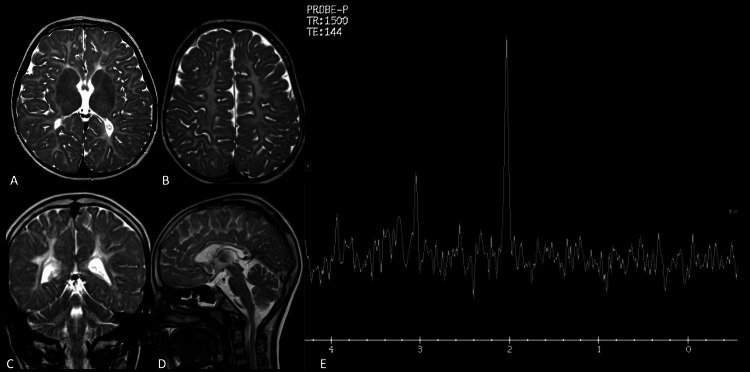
Salla's disease Confluent white matter T2 hyperintensity is visualized above (A) and at the level of the basal ganglia (B). The subcortical white matter is predominantly involved. Note the relatively hypointense basal ganglia and thalami on the axial T2-weighted image (B). The coronal T2-weighted image (C) shows symmetrical hyperintensity in the supratentorial and cerebellar white matter. The sagittal T2-weighted image (D) shows a thinned-out corpus callosum. Proton MRS at an intermediate TE (E) is remarkable for a prominent peak at 2.02 ppm, which is due to N-acetylneuraminic acid in this case of Salla's disease and closely resembles the NAA peak. TE: time to echo; NAA: N-acetyl aspartate
